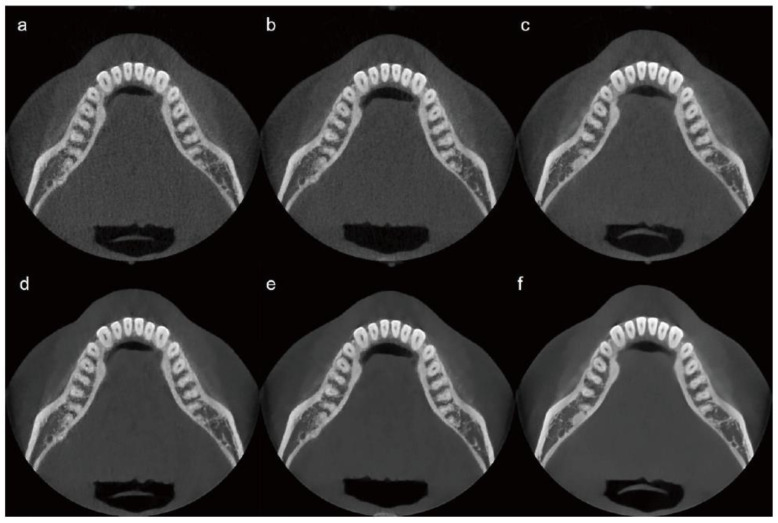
CBCT image sets categorized by radiation dose level and AI processing status. (a) 10% dose, raw image; (b) 20% dose, raw image; (c) 100% dose, raw image; (d) 10% dose, AI-processed image; (e) 20% dose, AI-processed image; (f) 100% dose, AI-processed image. AI, artificial intelligence; CBCT, cone-beam computed tomography.
